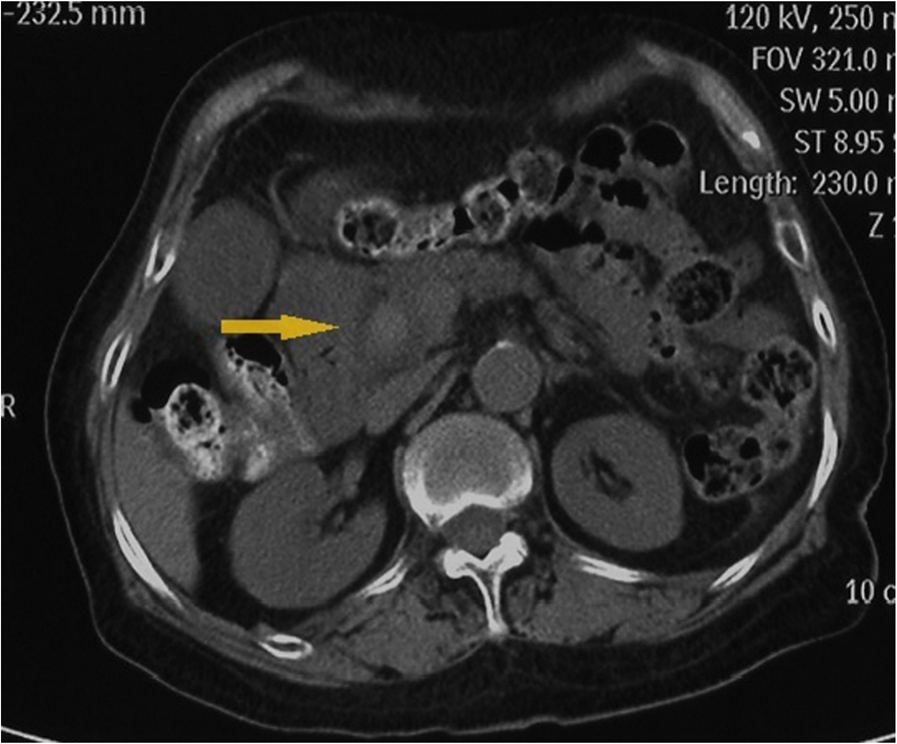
A high-density image of the middle CBD with a markedly dilated biliary tree on the second CT
